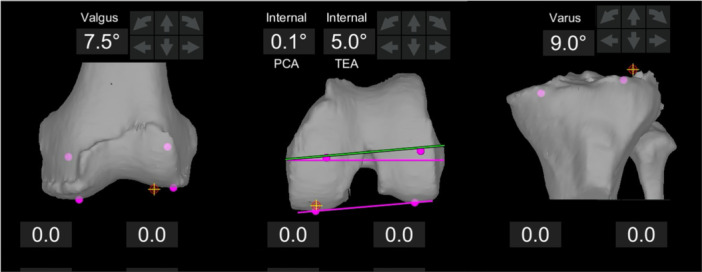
Intraoperative screenshot of the Mako (Mako Surgical Corp. [Stryker]) robotic system, displaying the CT‐based three‐dimensional joint line orientation of the femur and tibia in a left knee. Femoral and tibial coronal alignment, as well as femoral transverse orientation are obtained by equalizing medial and lateral bony resections. In this case, femoral mechanical angle is −7.5° (valgus), tibial mechanical angle is 9.0° (varus). Therefore, LDFA is 90° + (−7.5°) = 82.5° and MPTA is 90° − 9.0° = 81°. Conversely, PCA is in −5.0° (internal) rotation relative to TEA. CT, computed tomography; LDFA, lateral distal femoral angle; MPTA, medial proximal tibial angle; PCA, posterior condylar axis; TEA, transepicondylar axis.
